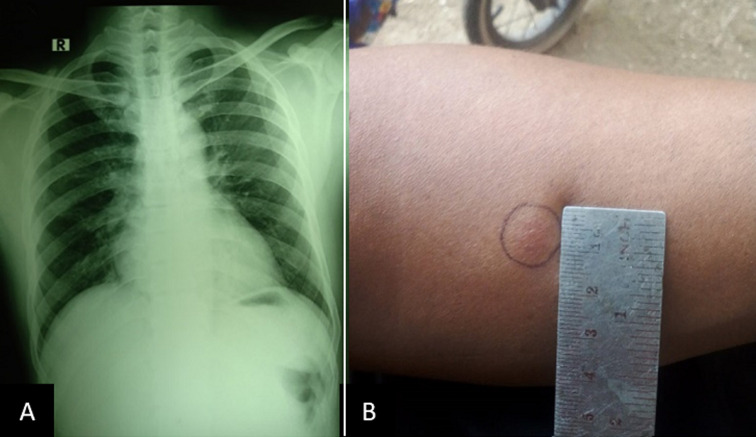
chest radiograph and Mantoux test: (A) normal result of chest radiograph; (B) negative result of Mantoux test
